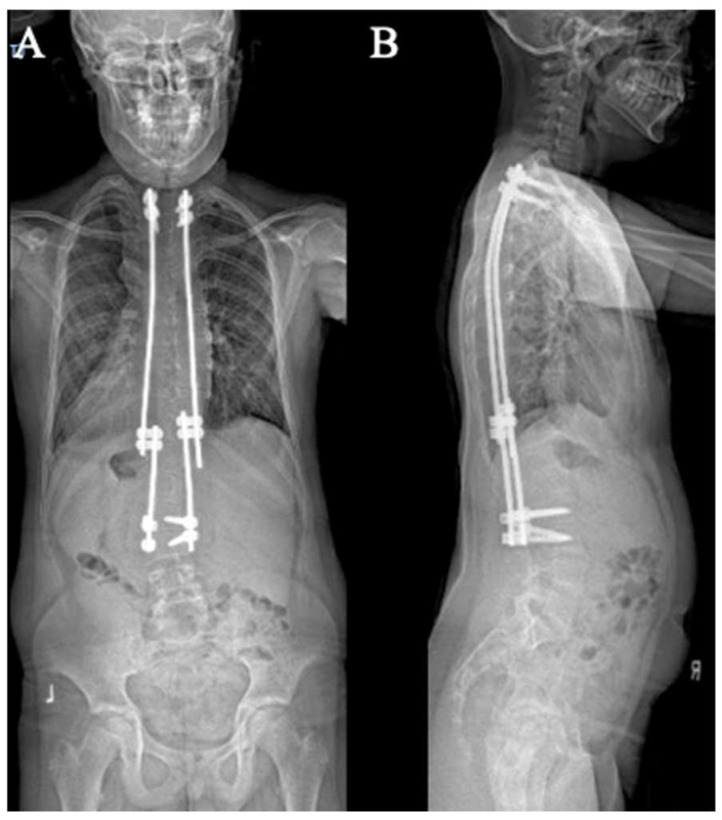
AP (A) and lateral (B) X-rays demonstrating the construct-to-construct internal distraction technique in a traditional growing rod construct.
